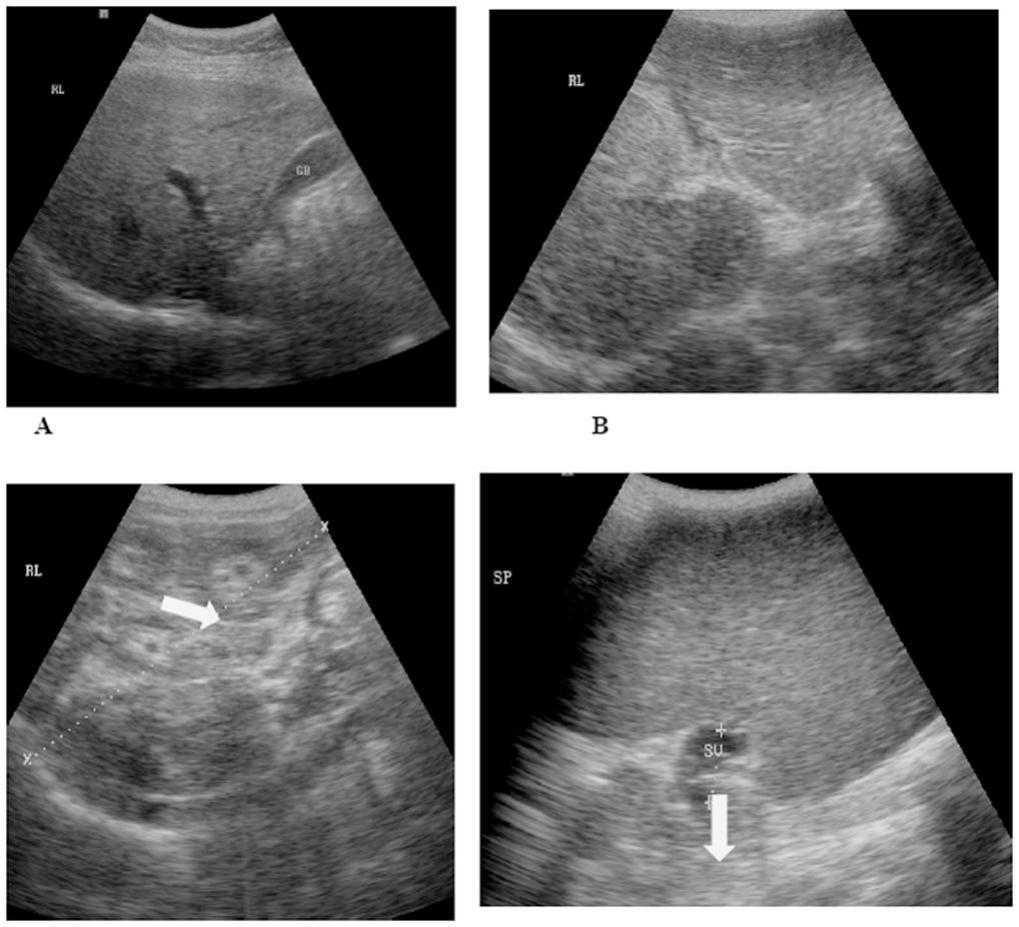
A, B, C, and D are representative ultrasound pictures that are seen in *S. japonicum* endemic areas. 1A shows a normal liver. 1B shows a liver with Grade II fibrosis (white arrow). 1C is Grade III fibrosis. The “Bull’s Eye” appearance is indicated by the white arrow in this image. Image 1D shows a very large spleen with dilated splenic vein (marked by white arrow).
